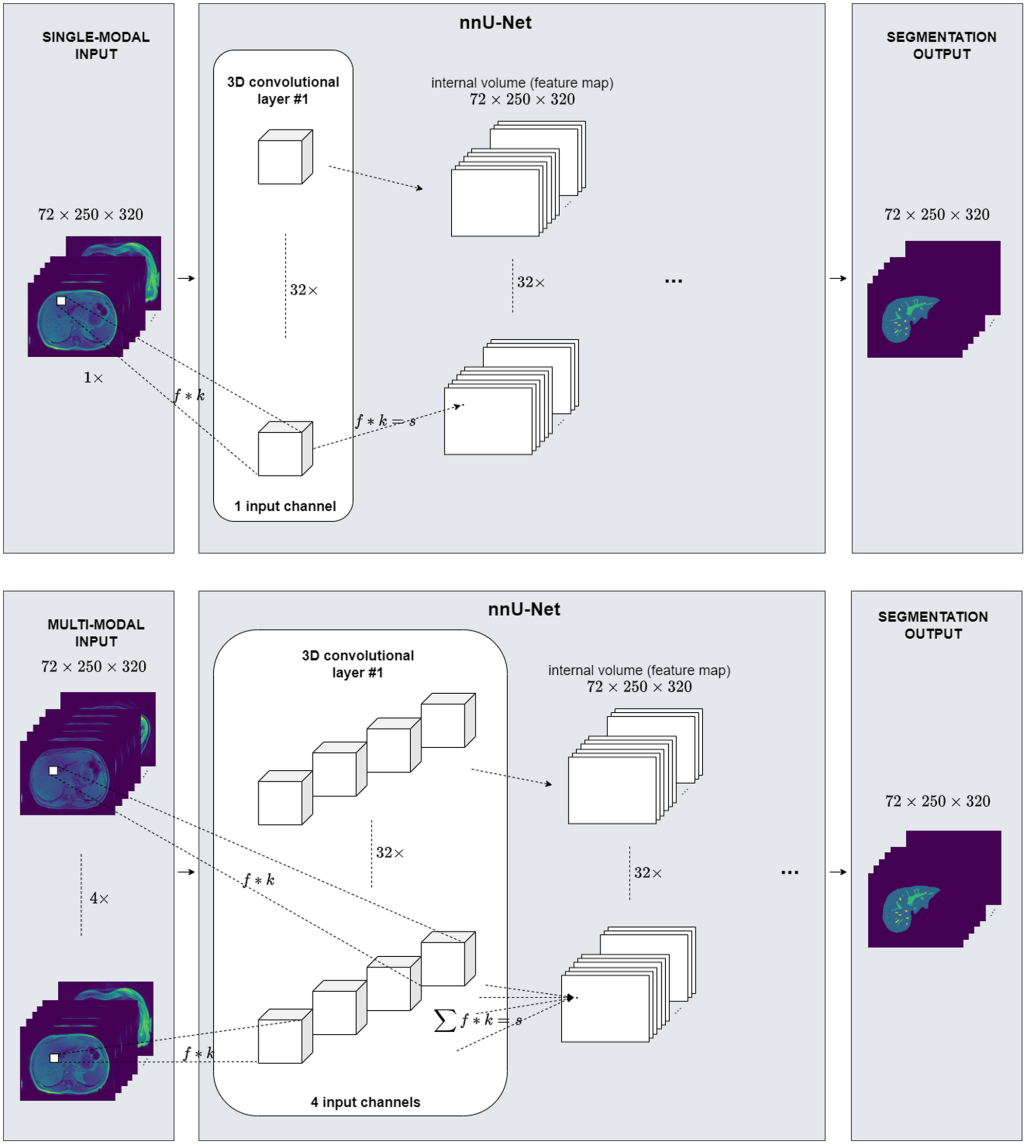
Processing of single-modal (top row) and multi-modal (bottom row) MRI sequence data at the input level of the 3D U-Net in use (nnU-Net^23^). The implementation uses 2D kernels at the first convolutional layer #1 since it treats the MRI sequences as anisotropic data.
